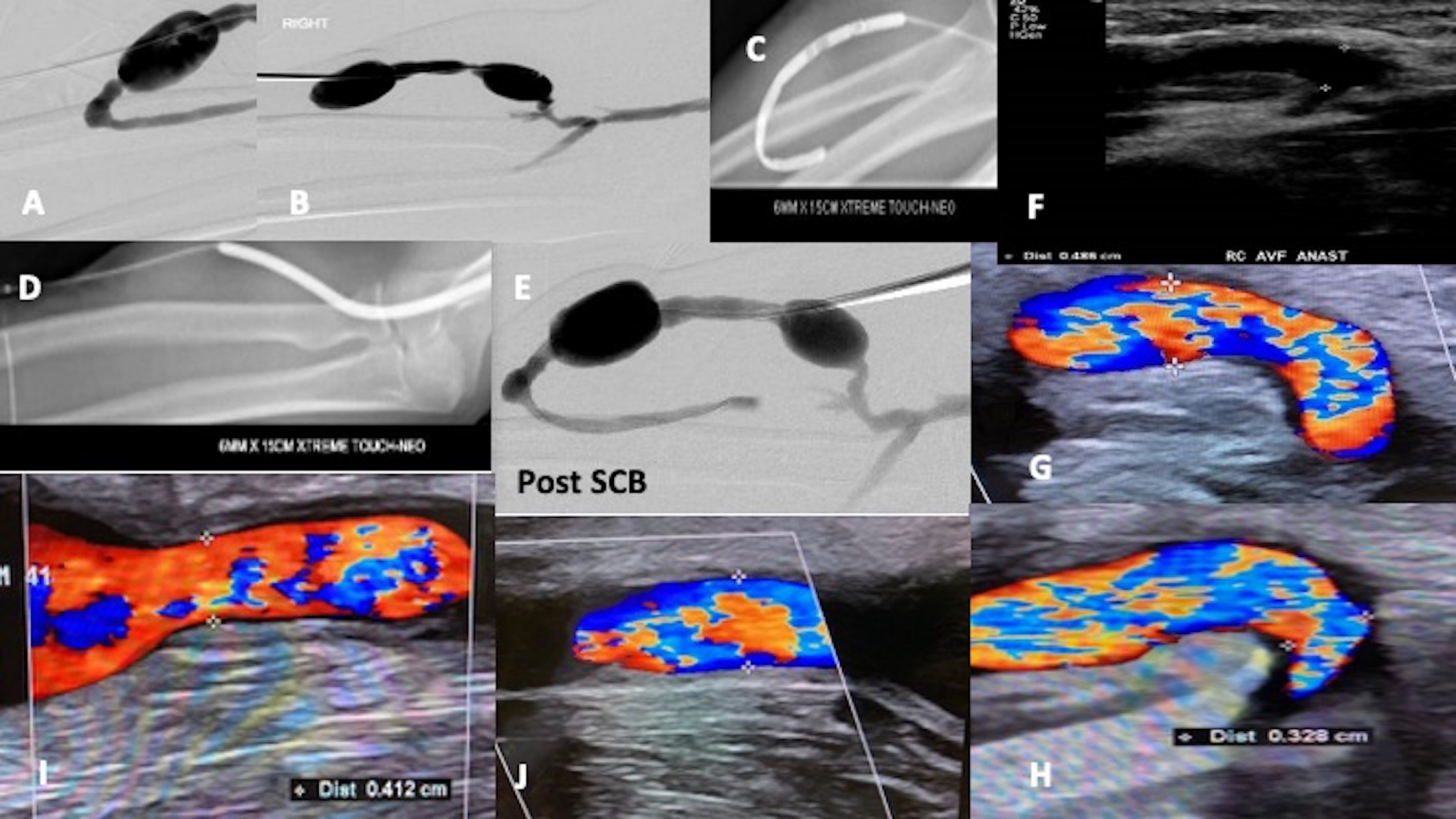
Case-study using sirolimus coated balloon. 74-year-old Malay male with a right mid-forearm fistula of 4.2 years maturity presenting with dropping access flow and having had CBA within 3 months prior (A) pre-plasty fistulogram showing a juxta-anastomotic stenosis (B) intra-aneurysmal segment stenosis and cephalic outflow vein stenosis located near antecubital fossa (C) & (D) use of sirolimus coated balloon drug elution post pre-dilatation with high pressure non-compliant balloons for 2 minutes (E) final fistulogram result with good luminal gain of both target lesions (F) baseline ultrasound post SCB at juxta-anastomosis (JAS) portion (G) Duplex ultrasound at 3 months post intervention at JAS and (H) 6 months post treatment at JAS (I) inter-aneurysmal segment portion of cephalic vein at 3 months and (J) same area at 6 months showing minimal change and access flow. One thing to note is that the NIH effect in the wall of the fistula was less pronounced at 3 and 6 months.
